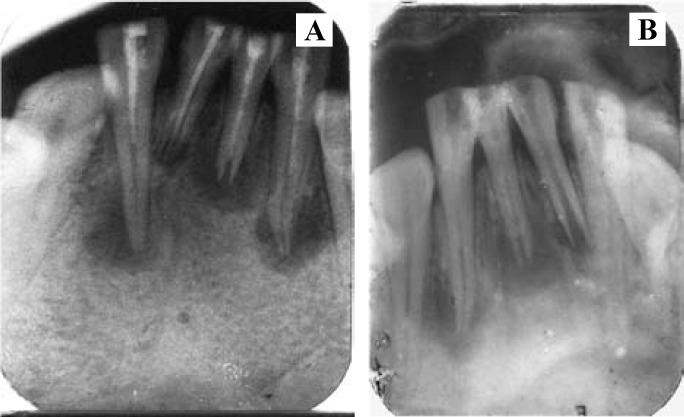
A. - Pre-operative Intraoral periapical radiograph. B. - After removal of old gutta-percha and sealer.
